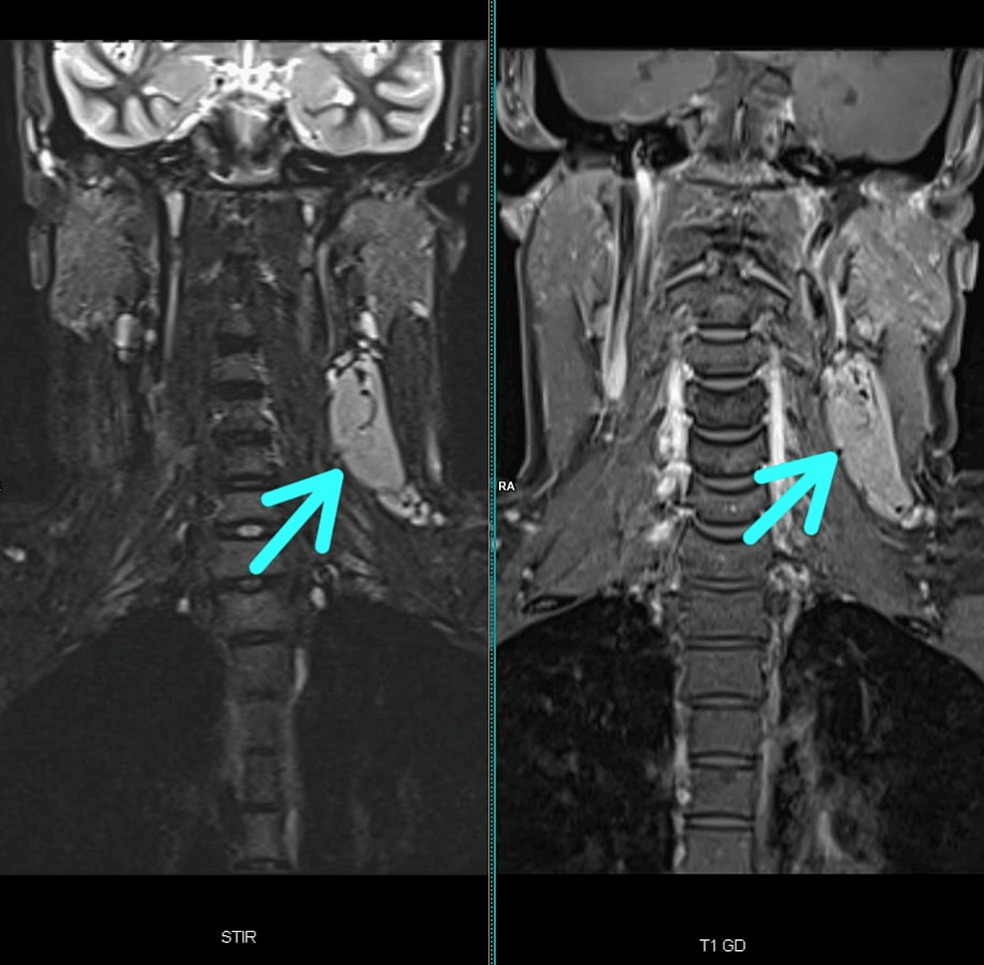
MRI showing an enlarged lymph node in the left III/IV cervical region. The lymph node appears as a hyperintense area (indicated by arrow), consistent with a solitary mass typical of unicentric Castleman’s Disease.
